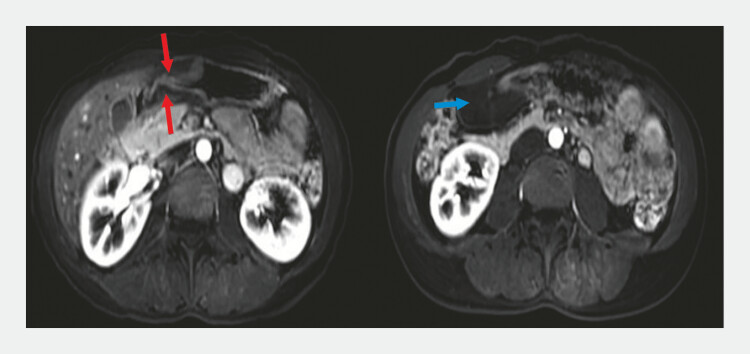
Abdominal enhanced magnetic resonance imaging scan. Submucosal lesions in the gastric antrum and descending duodenal bulb (red arrow). Cystic lesion between the perigastric and lesser omentum (blue arrow).
